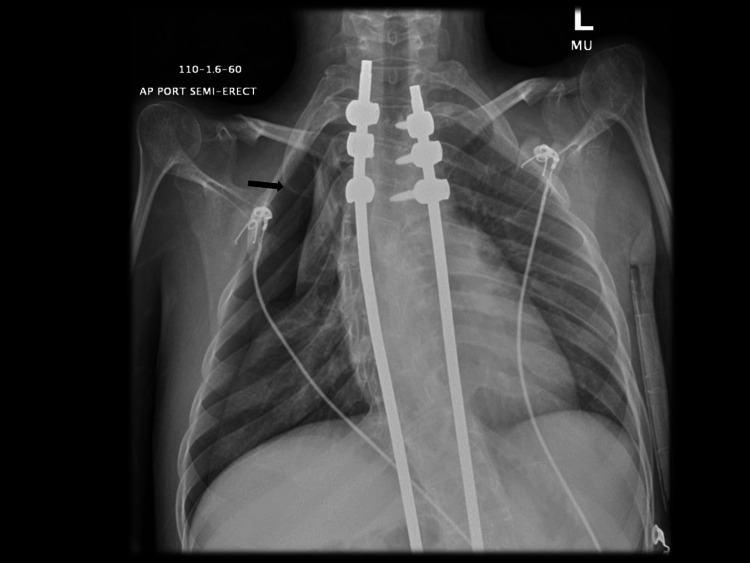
Chest radiograph of first pneumothorax located in the right upper lobe (black arrow).
